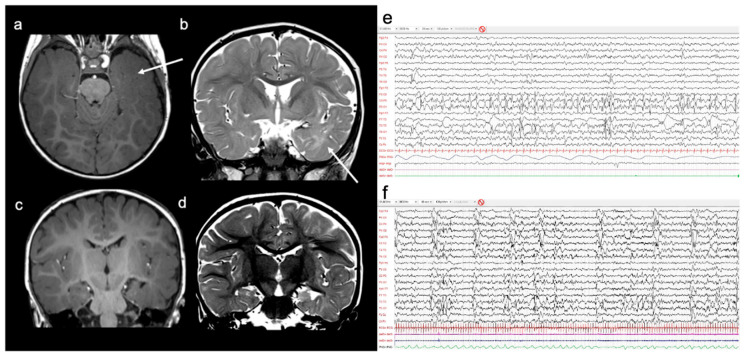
Magnetic resonance imaging T1-weighted images demonstrating left temporal pole volumetric reduction associated with blurring of the cortico-subcortical region (a, arrow) and hyperintensity of the white matter on T2-weighted sequences (b, arrow). There is incomplete hippocampal inversion on the left (c) associated with increased T2 signal (d). (e) EEG during Wakefulness shows continuous left temporo-occipital slow and epileptiform abnormalities, with sporadic contralateral expression. (f) Periodic complexes of slow waves after awakening associated with subtle eyes elevation, recorded in 60 s.
